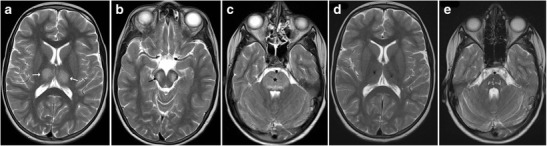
A 10-year-old patient with fever and altered sensorium: a–c Axial T2-weighted images show near symmetrical increased signal intensity in the medial thalami (*white arrow*), middle cerebral peduncles (*black arrow*) and pons (*asterisk*). The possibility of viral encephalitis, likely Japanese encephalitis, was considered. d–e Follow-up MRI: Axial T2-weighted images show hypointense foci (possibly remote haemorrhage) in the thalami with volume loss and an area of gliosis in the pons
